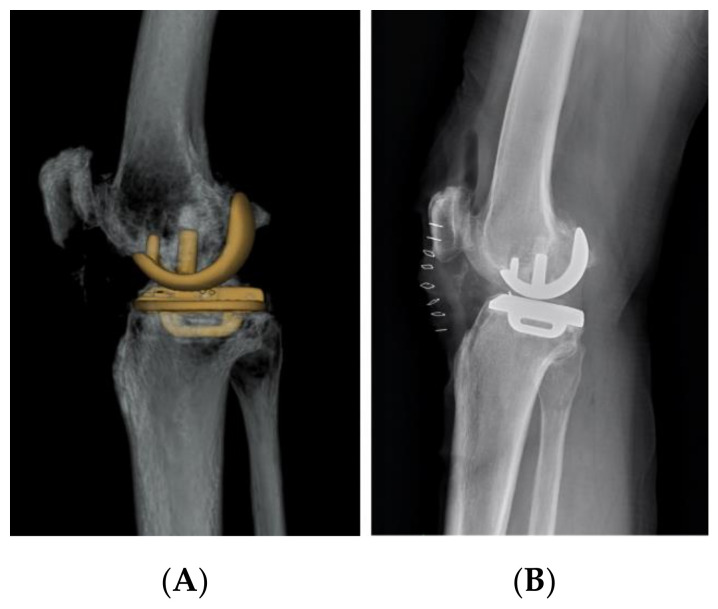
An example of ideal femoral component size of the knee lateral view and the same patient in CT scan showed a little overhang from posterior condyle bony contour. (A) 3D image. (B) Standard lateral view.
